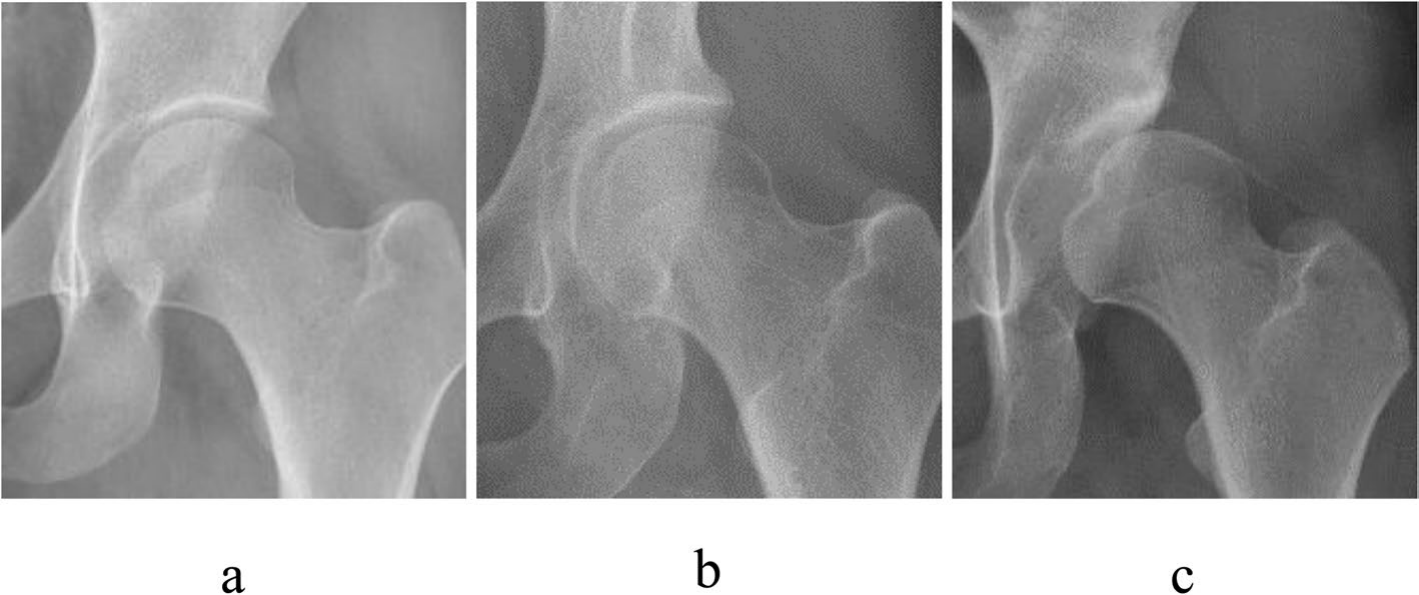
Radiographs (anteroposterior view) of the 3 candidates in present study. a. 35 years female who was normal acetabulum (CE angle was 30°). b. 39 years female who was borderline acetabular dysplasia (CE angle was 20°). c. 33 years female who was acetabular dysplasia (CE angle was 0°)
